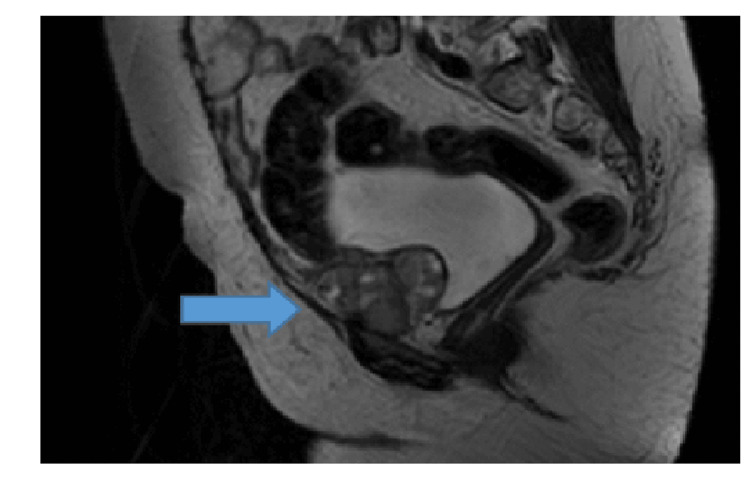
MRI pelvis T2 images showing multilobulated soft tissue masses in the retropubic fat anterior to the urinary bladder. There is no MRI evidence of invasion.
